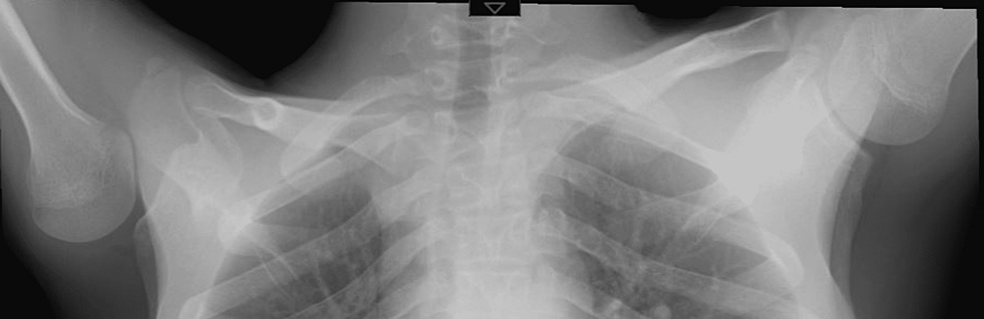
Standard X-ray showing an absence of congruence of the glenohumeral joint upon abduction of the arm
